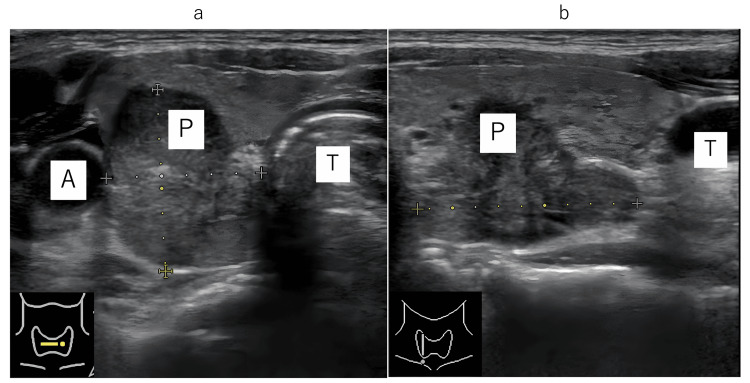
Head and neck ultrasound: (a) axial and (b) sagittal A: carotid artery; P: primary tumor; T: trachea
